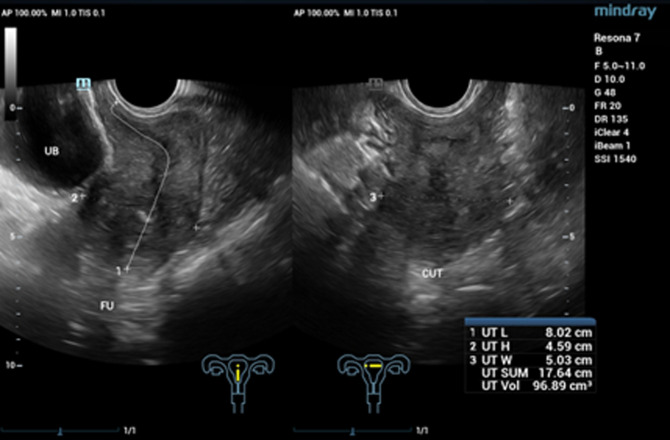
second ultrasound imaging of the patient after radiotherapy
